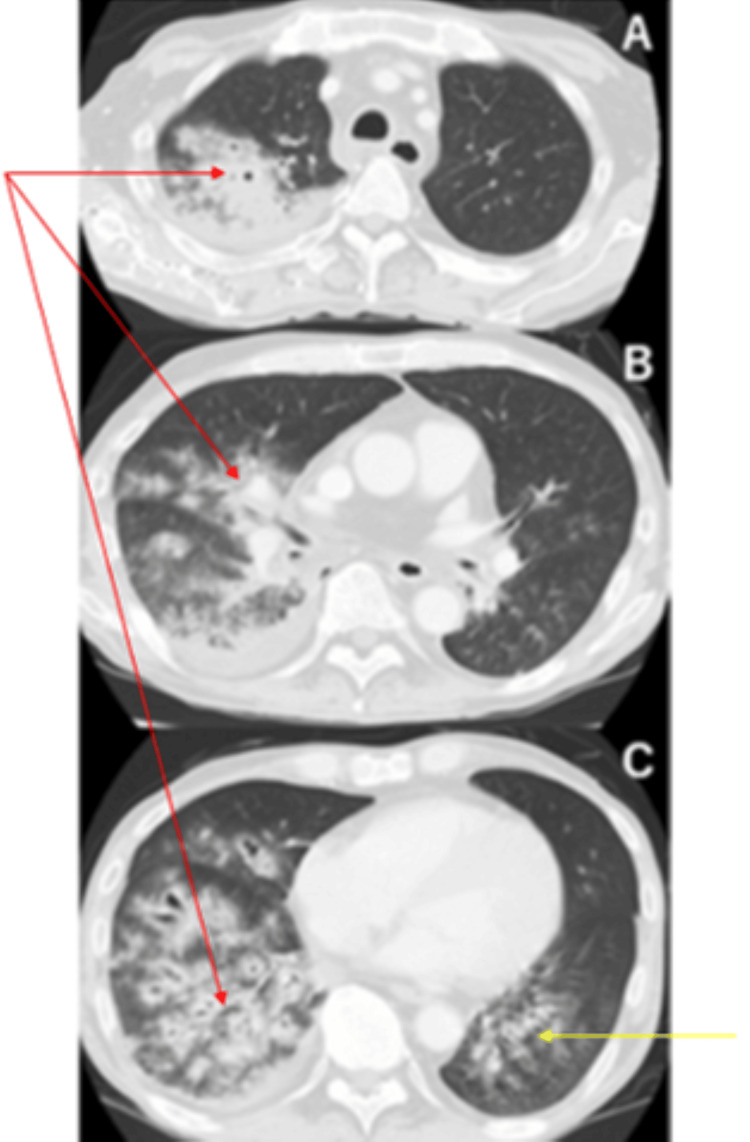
Follow-up axial CT on day x + 1 at (A) the upper lung fields, (B) the mid-thorax, and (C) the lower lung fields Follow-up axial CT on day x + 1 shows bilateral bronchocentric consolidation and ground-glass opacities. New bronchial dilation is visible, especially in the right lung (red arrow), consistent with necrotizing bronchopneumonia. A yellow arrow indicates a newly formed cavity within the area of consolidation, further supporting the diagnosis of necrosis.
